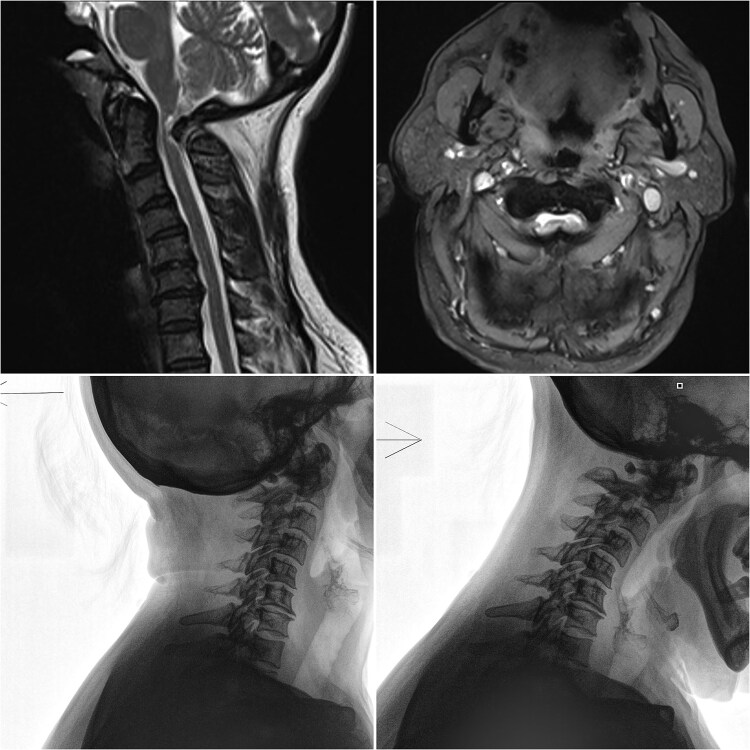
Sagittal and axial MRI, along with flexion/extension X-rays of the cervical spine, demonstrating anterior C1 subluxation, central canal narrowing, and myelopathy.
